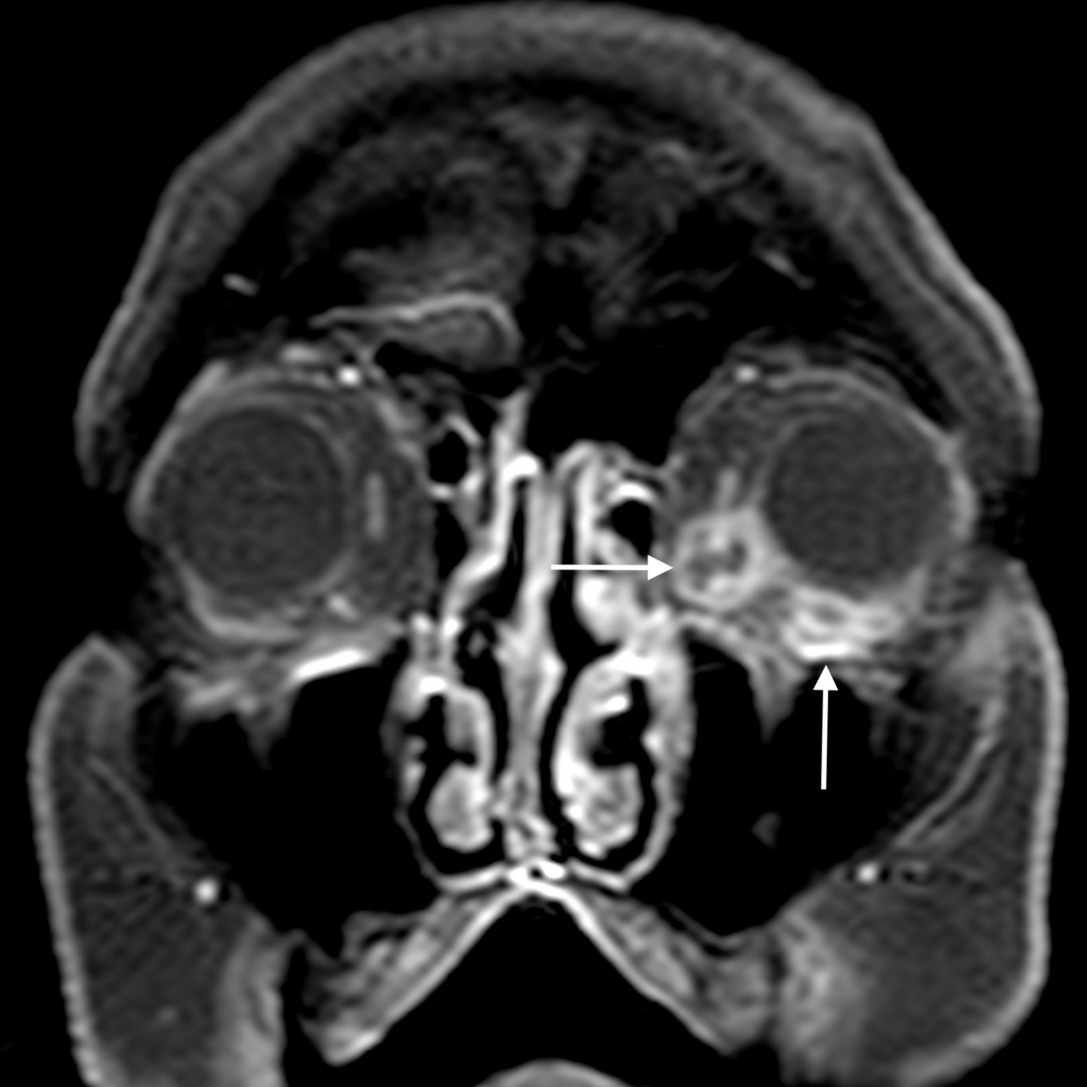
Orbital abscess: coronal post gadolinium T1WI shows peripheral enhancement of the lesions (arrows), indicative of abscess T1WI - T1-weighted image
